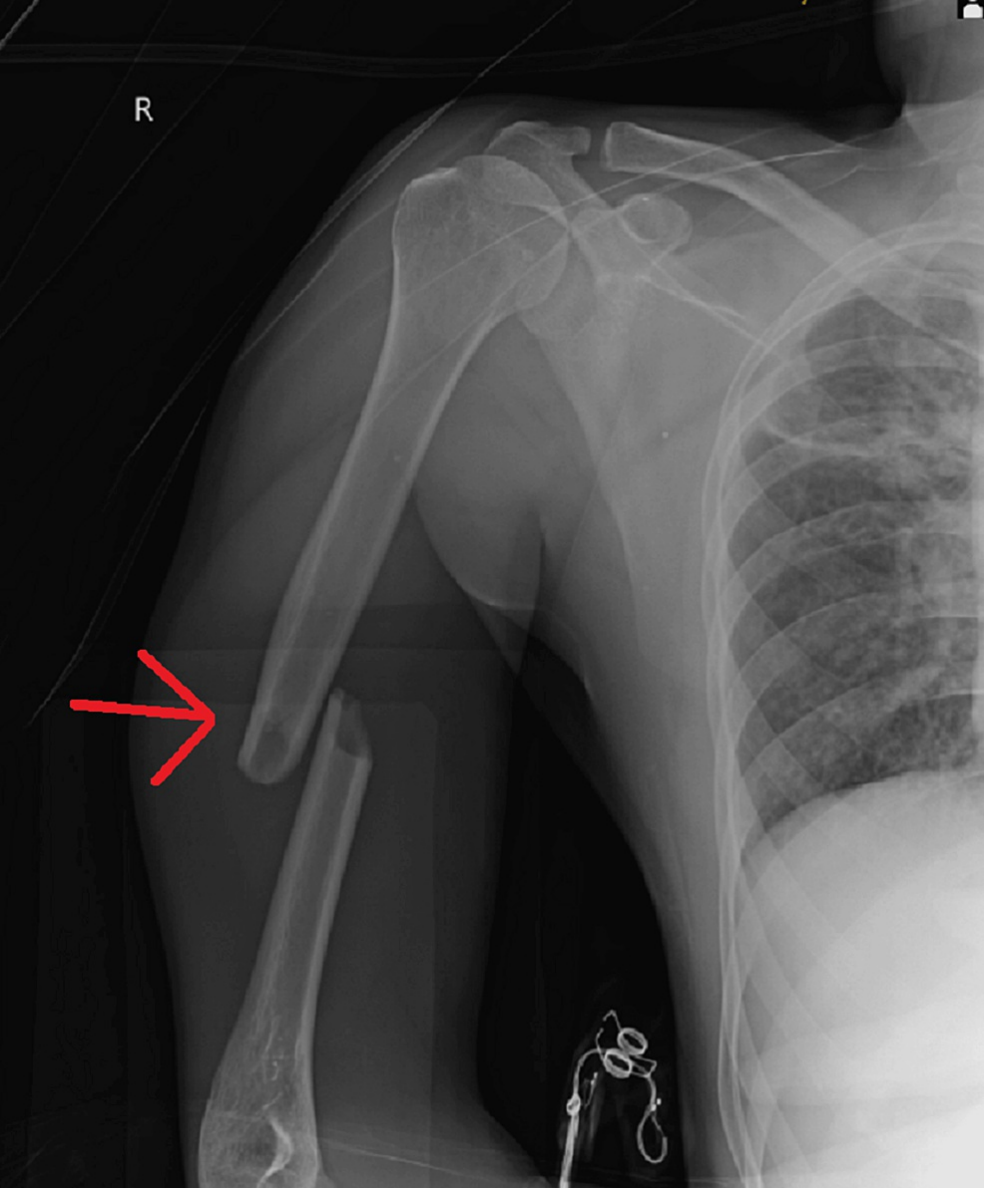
Lateral radiograph showing short oblique fracture mid-shaft region on right side
